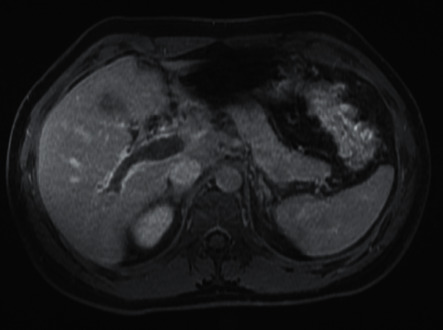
MRI of abdomen and pelvis with portal vein thrombosis.
